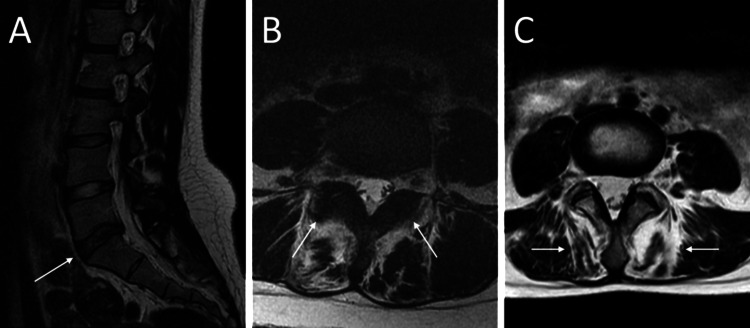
Degenerative changes observed on MRI. (A) Sagittal T2-weighted image showing disc degeneration at the L5-S1 level, characterized by an inhomogeneous signal and indistinct separation between the nucleus and annulus (white arrow), rated as Pfirrmann Grade 3. (B) Axial T2-weighted image demonstrating facet joint degeneration with pronounced osteophytes and severe osteoarthritis (white arrows), rated as Fujiwara Grade 4. (C) Axial T2-weighted image showing paraspinal muscle degeneration at the L5/S1 level with over 50% fatty infiltration and muscle atrophy (white arrows), assessed as Goutallier Grade 4.
